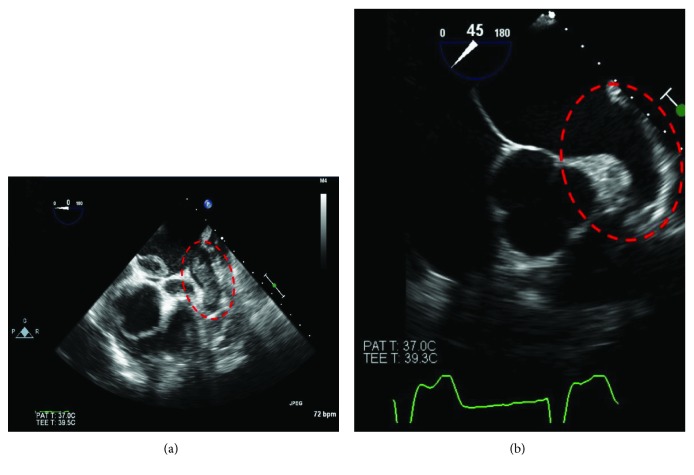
(a) The TEE transesophageal short axis view shows a large multilobulated mobile thrombus in the left atrial appendage. (b) TEE done 3 months later shows resolution of the thrombus.
